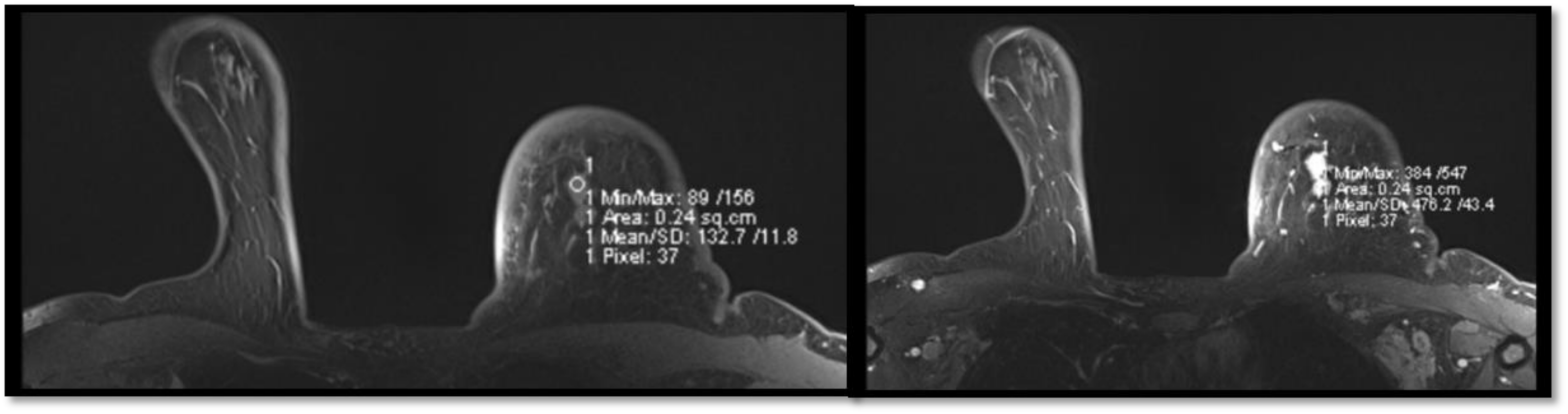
Axial T1-weighted Breast magnetic resonance imaging scans for a 41-year-old patient.
